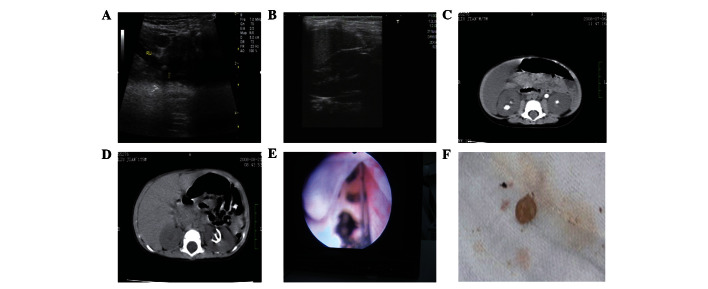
(A) Preoperative ultrasound. Stones (light dots, 10×6 mm) were observed in the uretero-pelvic junction. (B) Postoperative ultrasound. The stones had disappeared. No light dots were observed. (C) Preoperative computed tomography (CT). Multiple light dots were visible in the two kidneys. A strong light dot was observed in the left uretero-pelvic junction. (D) Postoperative CT. After indwelling epidural catheter placement, stones in the right kidney were discharged. In the left kidney, stones failed to be discharged. Therefore, open surgery was performed with the indwelling Double-J tube. (E) Cystoscopy indwelling ureteral stent. A deficit epidural catheter was inserted under a cystoscope. (F) Discharged stones. The stones were fragile and were easily crushed into a powder.
